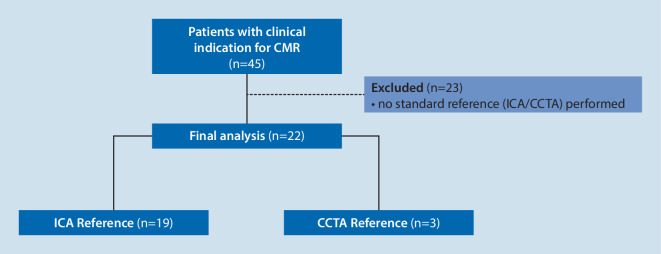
Algorithm for patient enrollment. *CMR* cardiovascular magnetic resonance imaging, *ICA* invasive coronary angiography, *ICA/CCTA* coronary computed tomography angiography
